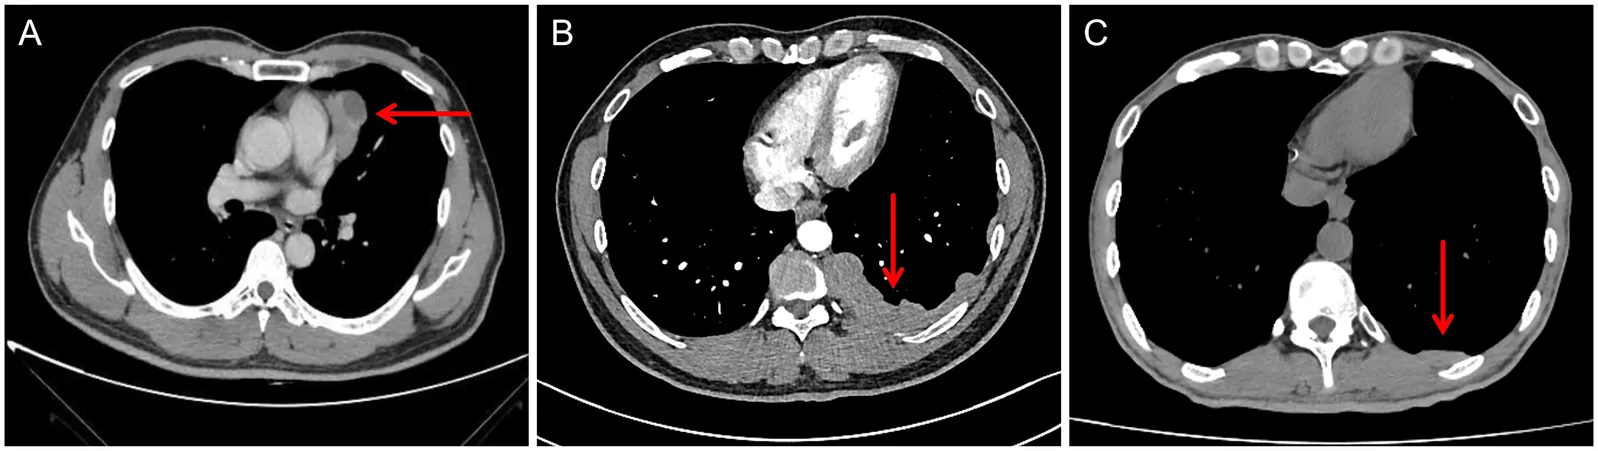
(A) (indicated by the arrow) shows a mass-like, irregularly-margined hypodense lesion measuring approximately 51 mm×35 mm in the anterior mediastinum adjacent to the pericardium, with an internal roundish low-density area, as revealed by preoperative axial contrast-enhanced chest CT. (B) (indicated by the arrow) shows significant thickening of the left pleura on pre-chemotherapy axial contrast-enhanced chest CT, which was considered metastatic. (C) (indicated by the arrow) shows a follow-up axial chest CT obtained after the completion of all chemotherapy cycles, demonstrating a marked reduction in both the degree of left pleural thickening and the extent of the lesion compared to the pre-chemotherapy findings.
